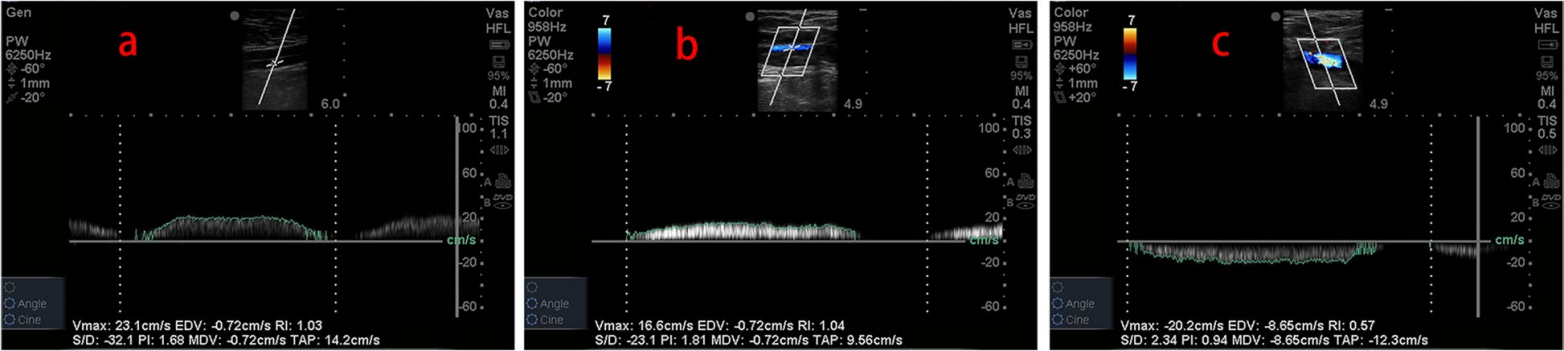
The Doppler sample volume cursor was positioned at the center of the venous lumen, and peak blood velocity and mean blood velocity were measured. a CFV point (proximal part of the junction of the superficial femoral vein and deep femoral vein). b GSV point (distal part of the junction of the GSV and CFV). c junction point (proximal part of the junction of the GSV and CFV)
